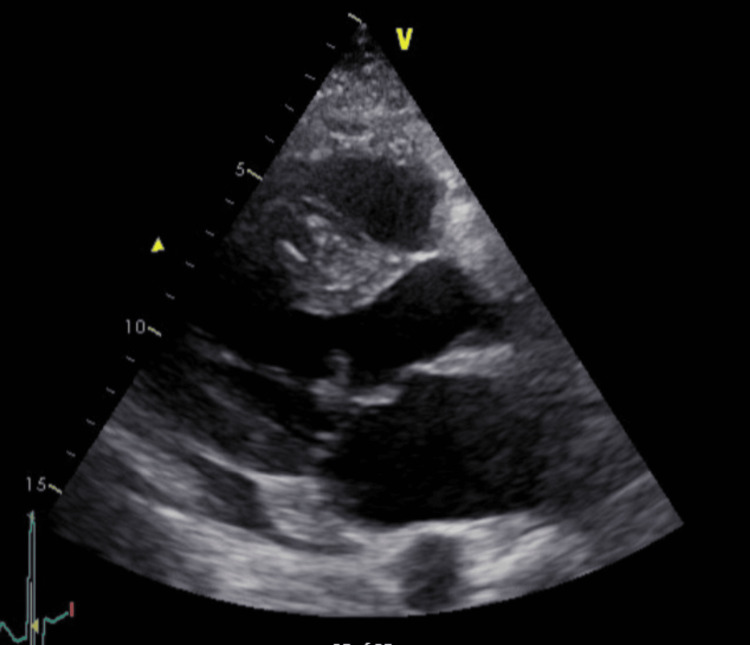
TTE showing resolution of mitral valve vegetation. TTE: transthoracic echocardiogram.
